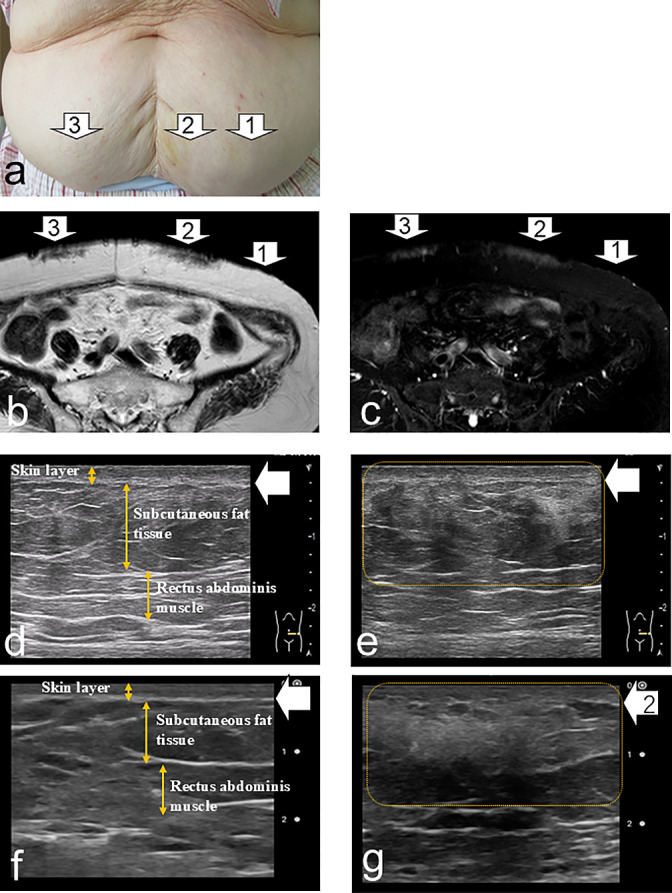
Subcutaneous induration and deep subcutaneous mass detected by visual inspection and palpation (Patient 12) a: No skin abnormalities were observed at a site with a low frequency of insulin injections (white arrow 1). Subcutaneous induration and a deep subcutaneous mass were identified at the insulin injection sites (white arrows 2 and 3). b: T2-weighted image of the lower left abdomen at a site with a low frequency of insulin injections (white arrow 1) showing no abnormalities. T2-weighted images of sites with subcutaneous induration and masses (white arrows 2 and 3) display low signal intensity in the areas indicated by the arrows compared with the surrounding subcutaneous fat tissue. c: Fat-suppressed T2-weighted image of the lower left abdomen at a site with a low frequency of insulin injections (white arrow 1) showing no abnormalities. Fat-suppressed T2-weighted images of sites with subcutaneous induration and masses (white arrows 2 and 3) demonstrate high signal intensity in the regions indicated by the arrows compared with the surrounding subcutaneous fat tissue. d: High-resolution ultrasound image of the lower left abdomen at a site with a low frequency of insulin injections (white arrow 1) showing a well-defined boundary between the dermis and subcutaneous fat. The dermis (white arrow) is clearly visualized, and the echogenicity of the subcutaneous fat is within normal limits. e: High-resolution ultrasound image of the lower left abdomen at a site with subcutaneous induration and mass (white arrow 2) showing an indistinct dermis–subcutaneous fat boundary (white arrow) and altered echogenicity of the subcutaneous fat compared with the surrounding tissue (yellow dotted line). f: Pocket-sized ultrasound image of the lower left abdomen at a site with a low frequency of insulin injections (white arrow 1) showing a well-defined dermis–subcutaneous fat boundary. The dermal layer (white arrow) is clearly visualized, and the echogenicity of the subcutaneous fat appears normal. g: Pocket-sized ultrasound image of the lower left abdomen at a site with subcutaneous induration and mass (white arrow 2) showing an indistinct dermis–subcutaneous fat boundary (white arrow) and altered echogenicity of the subcutaneous fat relative to the surrounding tissue (yellow dotted line).
